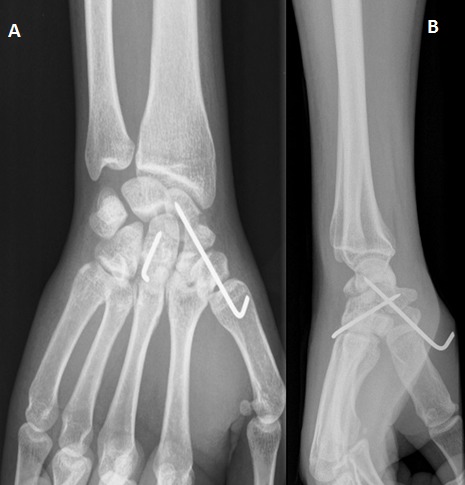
Radiographie du poignet droit de face et de profil: embrochage scapho-capital (A,B)
